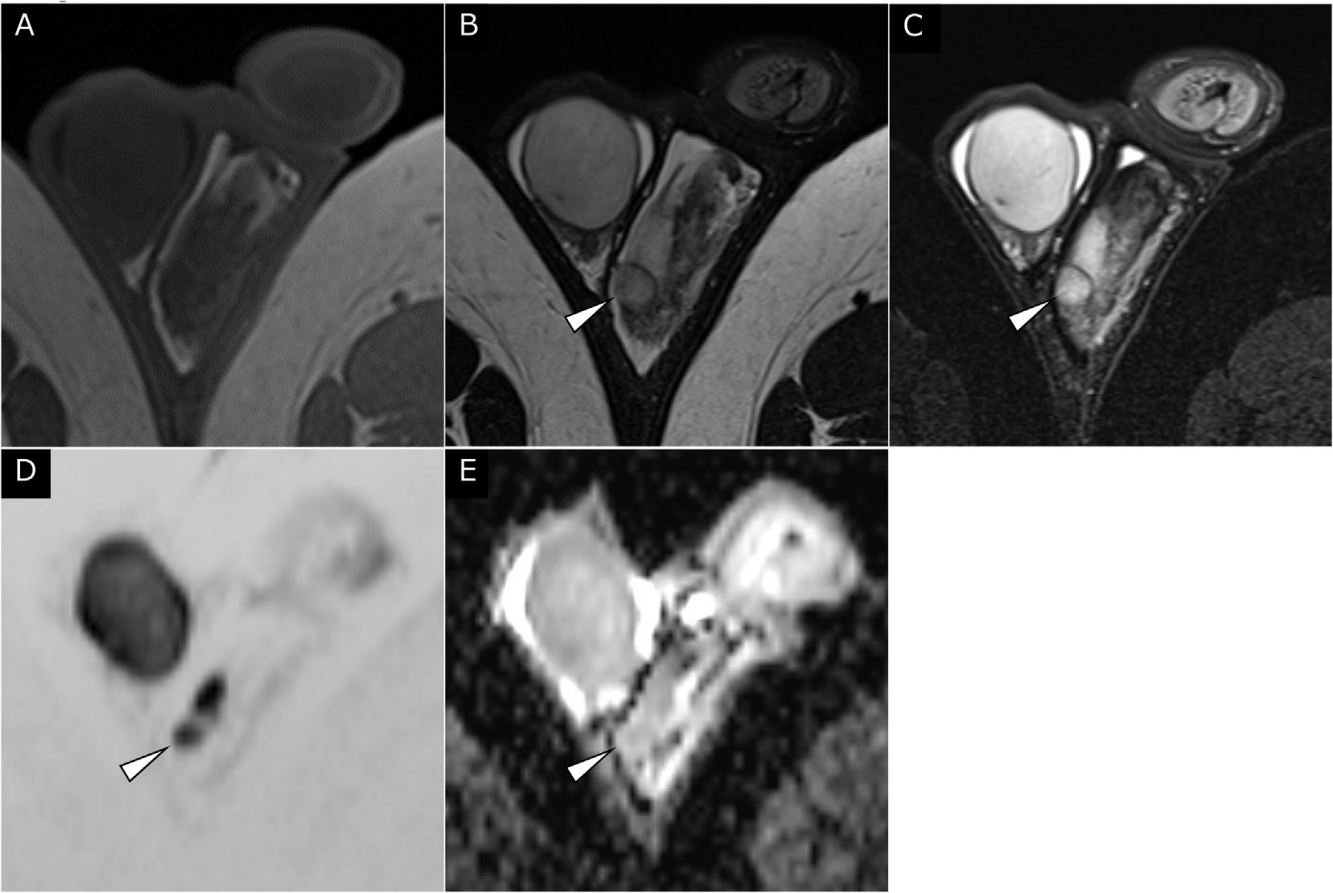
Axial MRI of the left scrotal mass. (A) T1WI failed to demonstrate the tumor. A well-defined slightly inhomogeneous 10-mm mass of high signal intensity (arrowheads) was present at the periphery of the left testis on (B) T2WI and (C) fat-suppressed T2WI. (D, E) On DWI, the mass (arrowheads) showed diffusion restriction comparable to that of the normal testis (apparent diffusion coefficient of the mass: 1.1 × 10^−3^ mm^2^/s).
